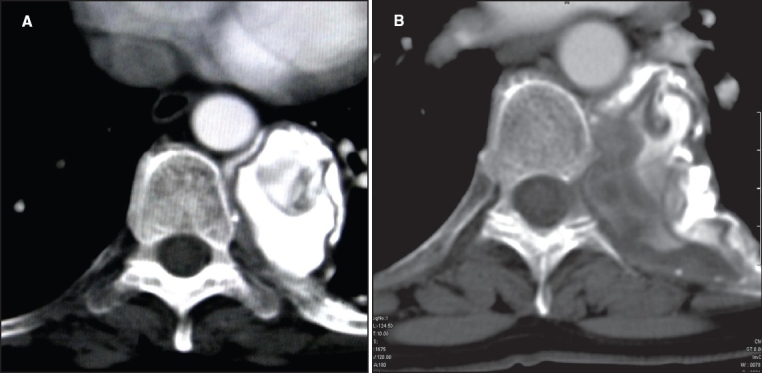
Axial contrast-enhanced CT of the chest
